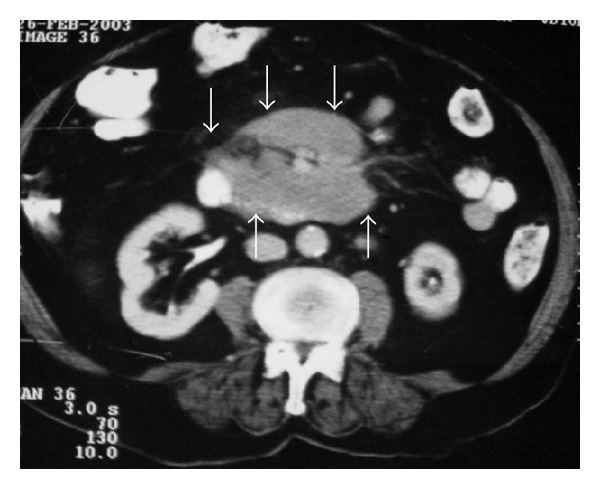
CT of the abdomen demonstrates lymph nodes involving mesenteric vessels (sandwich sign) anterior to aorta and inferior to vena cava in a patient with lymphoma (arrows).
